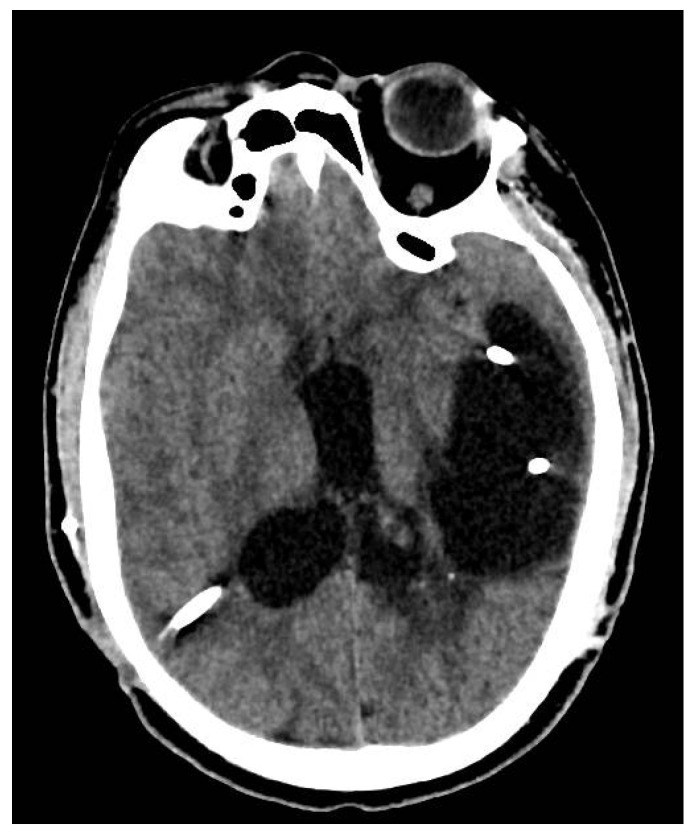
Cerebral CT scan—left temporal cystic cavity of 77/48 mm; similar infratentorial image of 29/19 mm in the axial plane, adjacent to the pons; ventricular drain tubes at the level of the body of left lateral ventricle, body and posterior horn of the right lateral ventricle.
